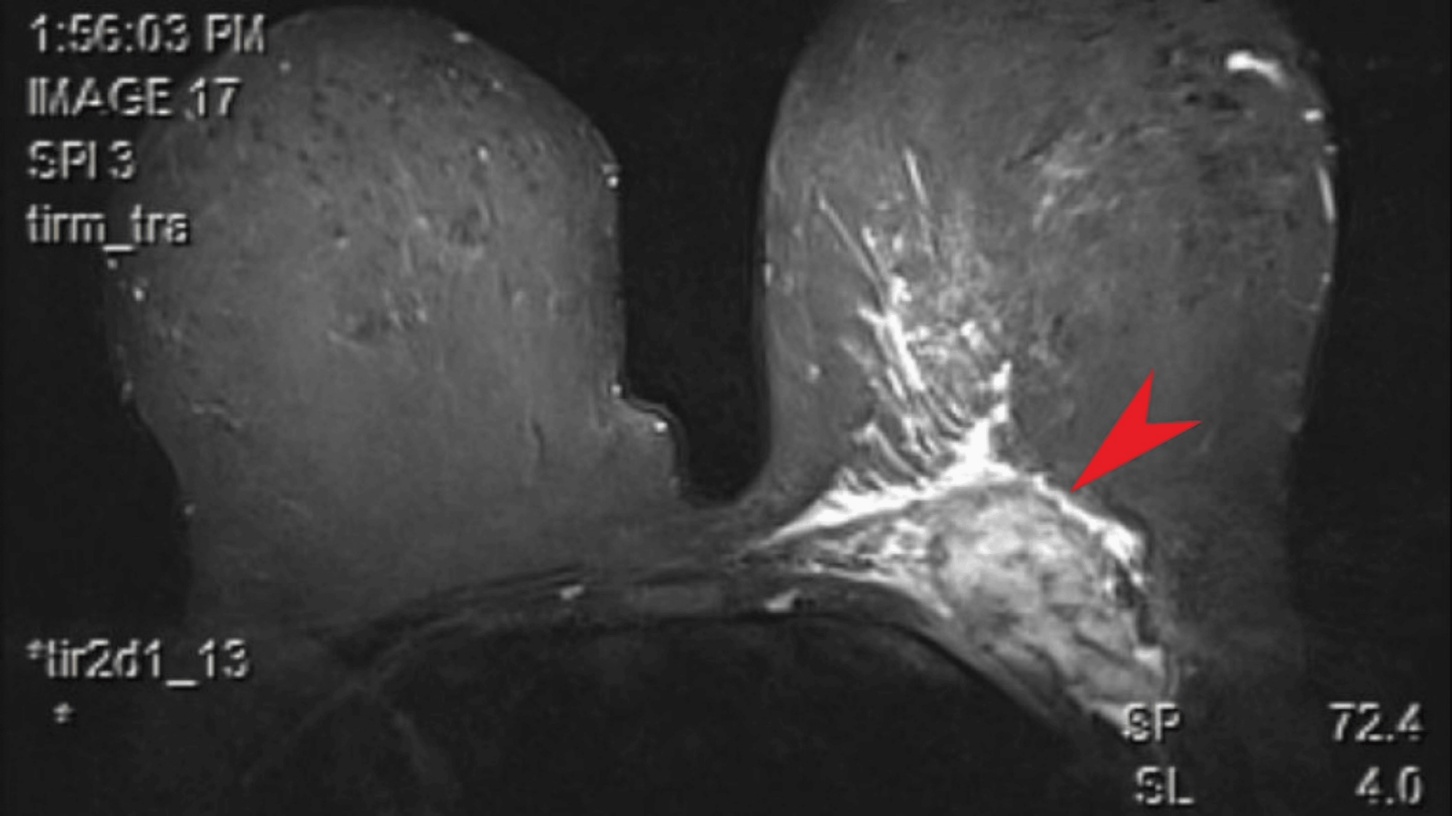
Breast Magnetic Resonance Imaging In the requested follow-up imaging for the tissue involvement of the lesion, involvement of the soft tissue and encapsulated nature of the mass (red arrow), with protrusions to the breast itself, can be seen.
